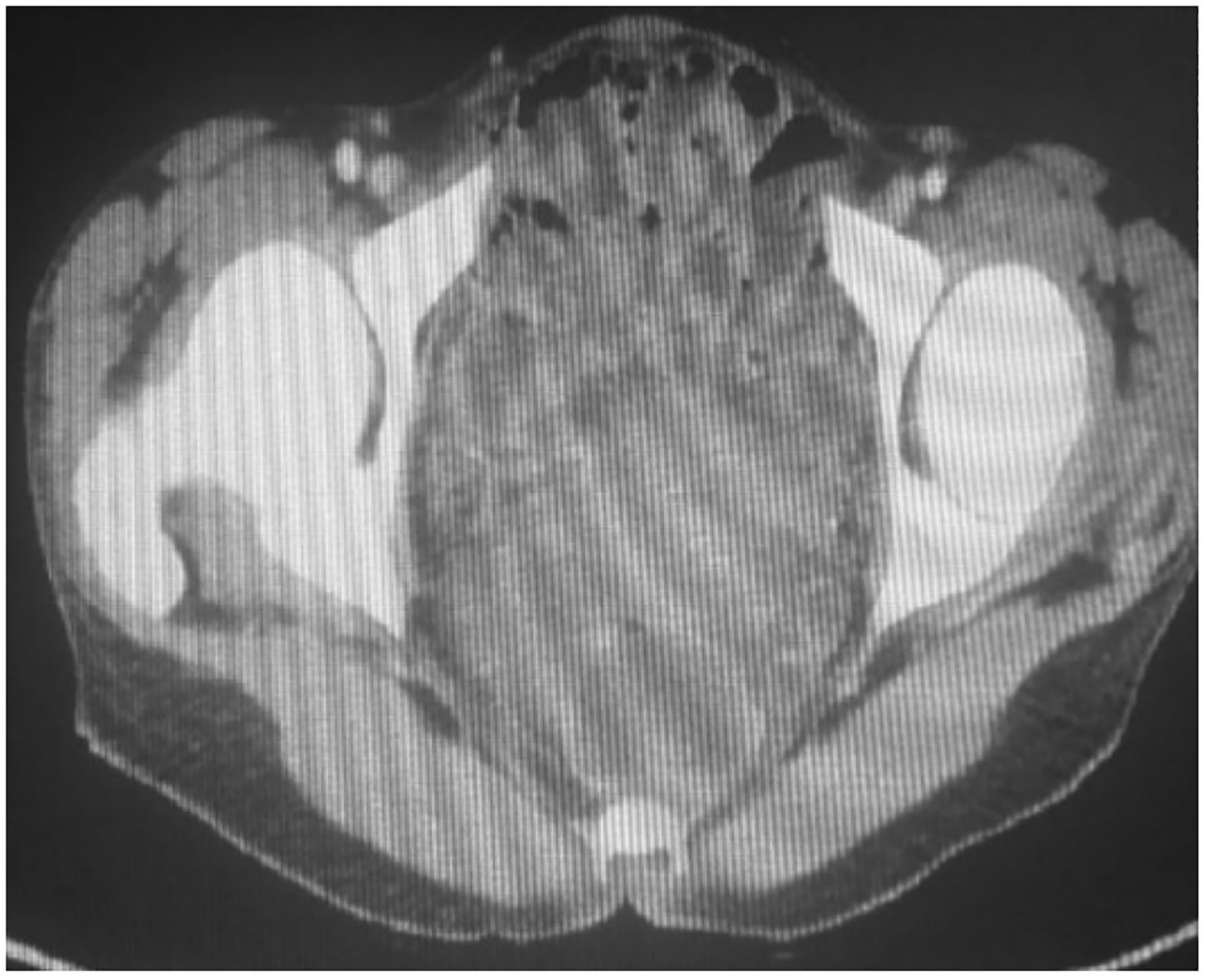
CT scan of the abdomen revealed mildly dilated, fluid‐filled small bowel loops with a fluid plume in the colon surrounded by air bubbles.
